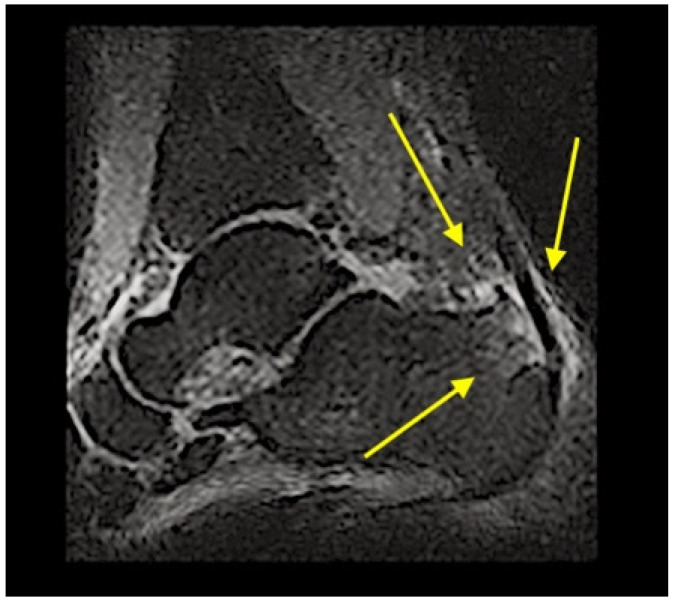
Magnetic resonance Imaging: The yellow arrows indicate: the degenerative changes in the Achilles tendon, bony oedema and the presence of retrocalcaneal bursitis, tendonitis phenomena and spongious oedema in the insertional region of Achilles tendon in the calcaneus.
